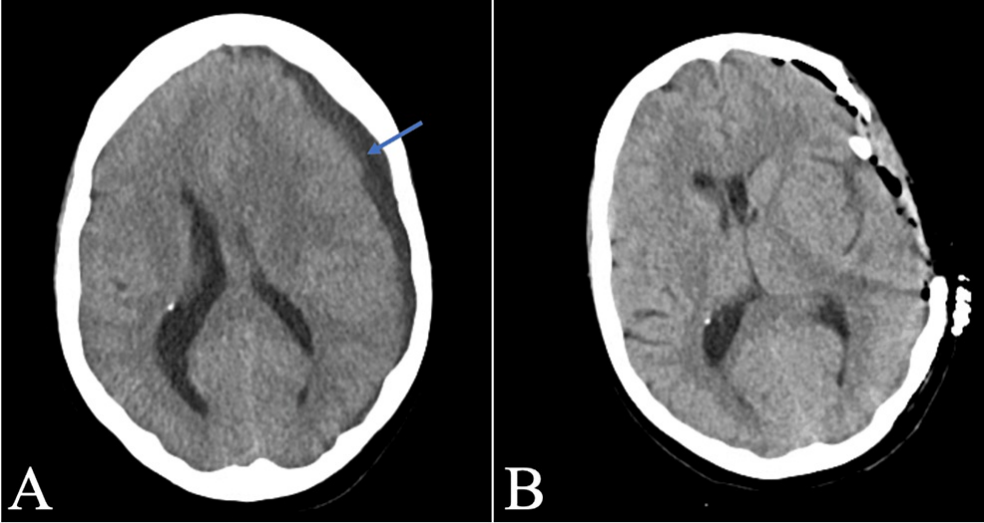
Radiographic images of a non-enhancing subdural empyema in a 42-year-old female with a remote history of seizure disorder and congenital deafness (A) Preoperative axial view of a non-contrast CT of the head showing an 8.8 mm low-density subdural fluid collection identified at the left frontotemporal and parietal levels. There is mass effect with effacement of sulci and effacement of the left lateral ventricle level of the frontal horn. There is a left-to-right midline subfalcine shift of 7 mm. Non-enhancing subdural collection is outlined with a blue arrow. (B)  Axial views of a post-operative non-contrast CT of the head, two hours post-operative, demonstrating a left-sided decompressive craniectomy and interval resolution of the previous subdural collection, with left-sided extra-axial drainage catheter in place, expected post-operative pneumocephalus, and trace extra-axial blood products. CT, computed tomography
